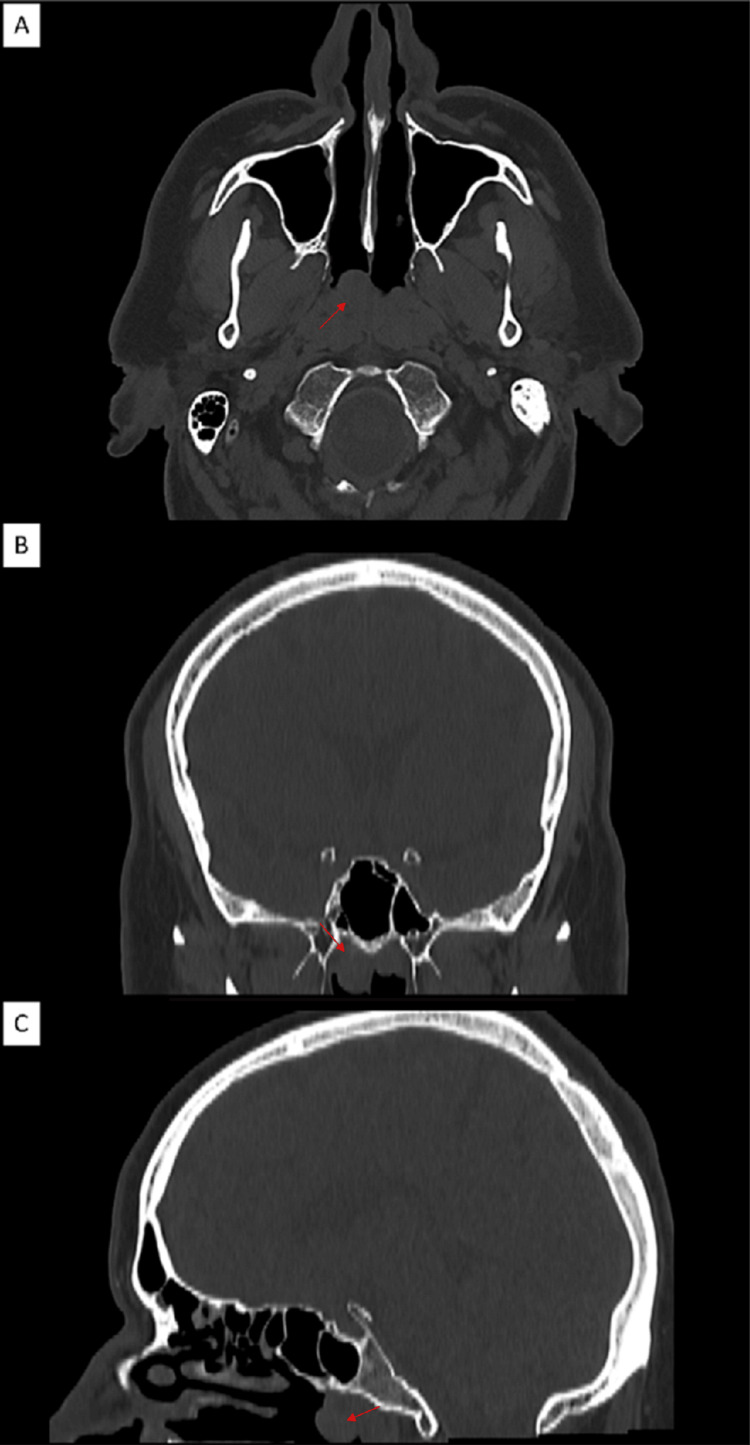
Computed tomography of a symptomatic Tornwaldt cyst. A. Axial view. B. Coronal view. C. Sagittal view. Red arrows show a well-rounded lesion in the nasopharynx.
